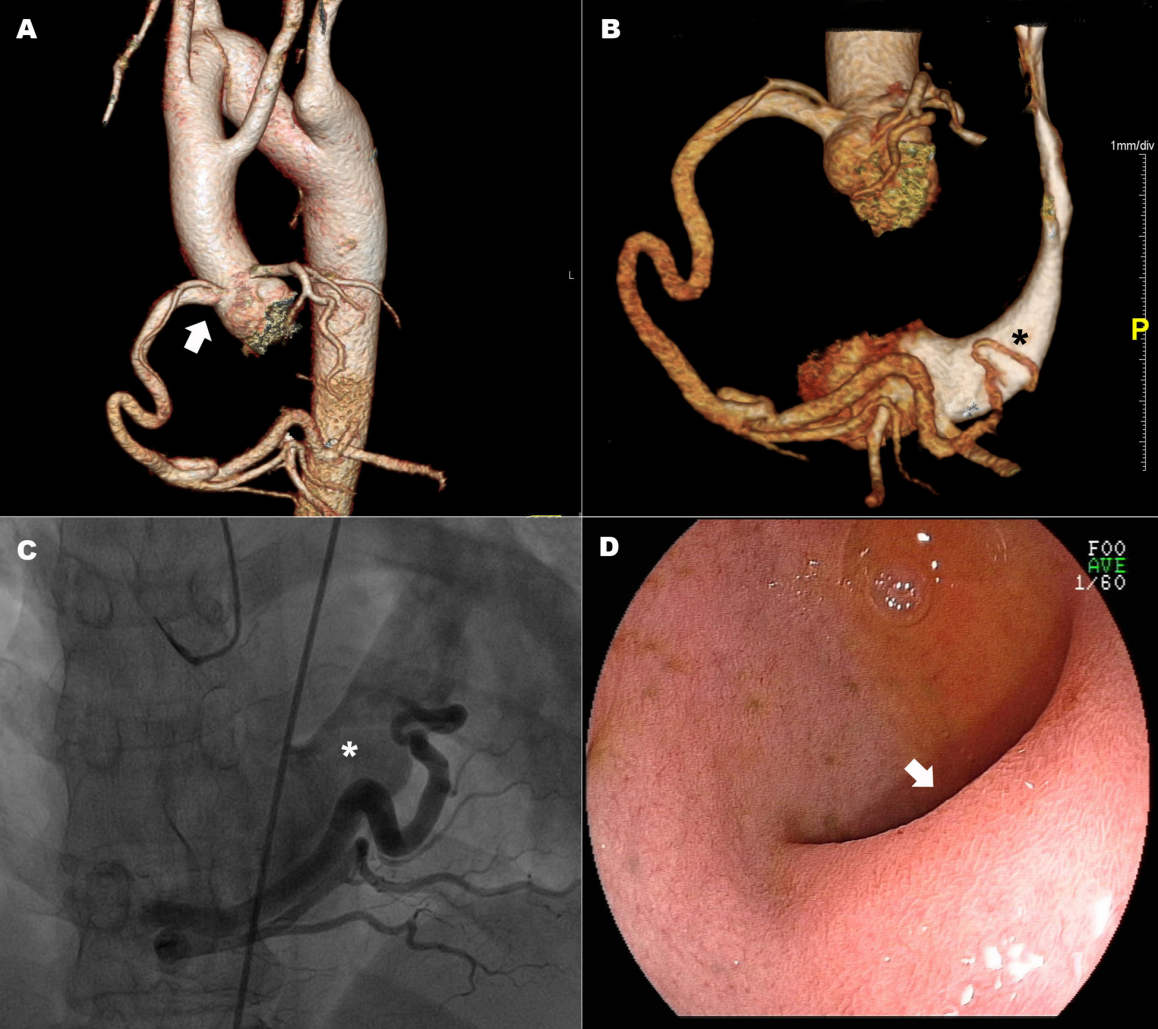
Combined vascular anomalies of the right-sided aortic arch. As evident in CT angiography, both coronary arteries arise above the sinus of Valsalva (A, arrow) and distal right coronary artery drains directly into the coronary sinus (B, *). The coronary artery-coronary sinus fistula was confirmed by angiography (C). Esophagus was compressed by a Kommerell’s diverticulum as apparent in esophagogastroduodenoscopy (D, arrow).
